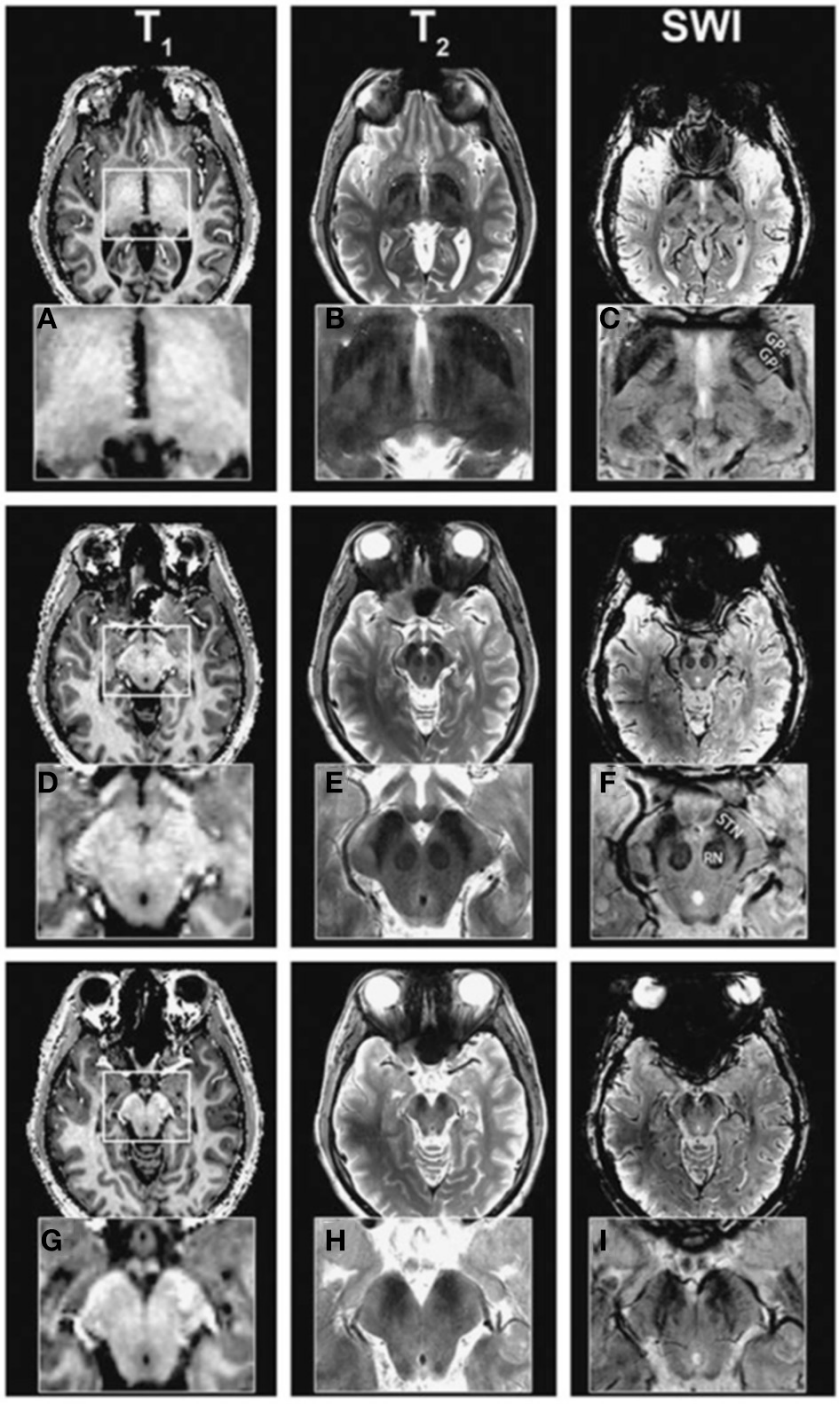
Ultra-high field (7T) T1-weighted (A,D,G), T2-weighted (B,E,H), and susceptibility-weighted (C,F,I) images at different levels. Adopted with permission from Abosch et al. (2010). The susceptibility-weighted images show the highest detail followed by the T2-weighted images.
